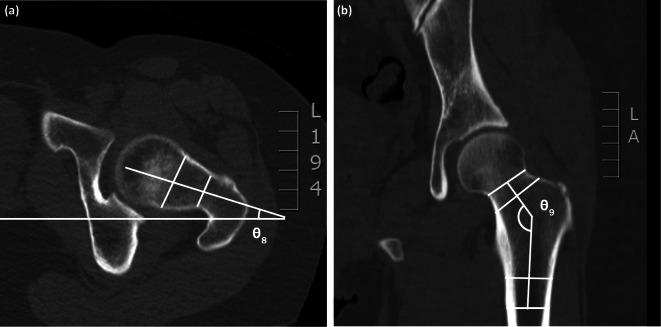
(A) Parameters used to measure coxa valga and the femoral anteversion. θ9 is femoral neck anteversion (FAV) described by Perreira AC. This parameter was measured on the axial images of CT scan. FAV was measured as the angle between a line bisecting the long axis of the femoral neck (from the center of the femoral head to center of the femoral neck) and a line parallel to the distal femoral posterior condylar axis. (B) Parameters used to measure coxa valga and the femoral anteversion. θ10 is the neck shaft angle (NSA). This parameter was measured on the coronal images of CT scan. NSA was measured as the angle between a line bisecting the long axis of the femoral neck and a line bisecting the long axis of the femoral shaft
